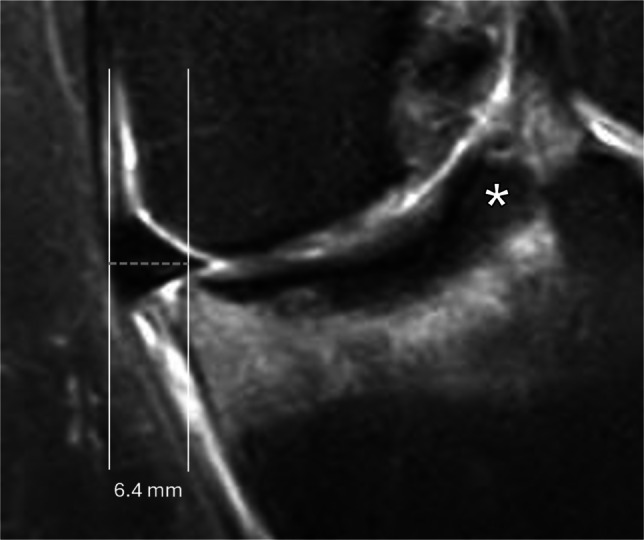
Pathological extrusion on the medial meniscus in a 69-year-old woman with complete radial tear of the posterior root of the medial meniscus and subchondral insufficiency fracture of the medial tibial plateau. Coronal proton-density-weighted fat-suppressed MRI depicts measurement of extrusion performed on the image with the greatest volume of the medial tibial spine (*), as a distance between peripheral chondroosseous margin of the tibia and outer edge of the meniscus
